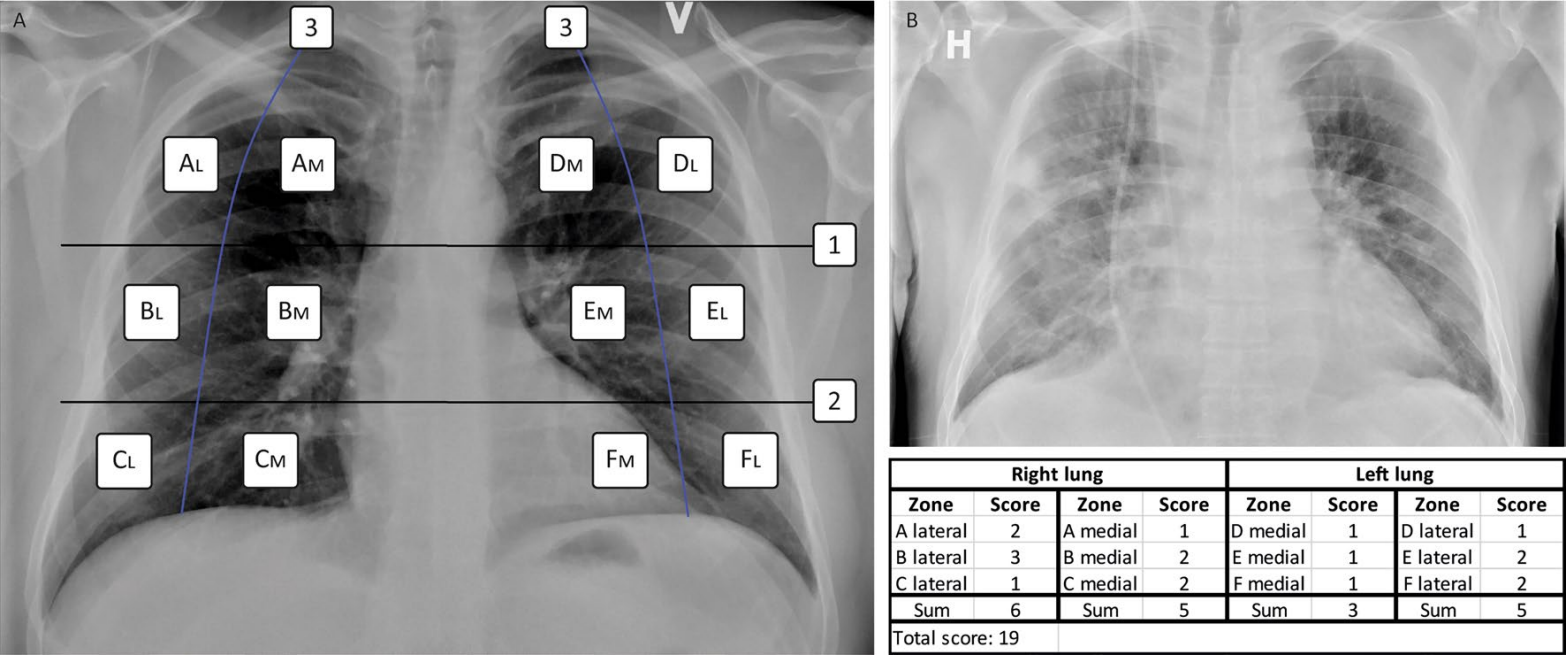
(A) The MBrixia scoring system. Box 1 and 2 mark the horizontal division corresponding to the inferior wall of the aortic wall, and the inferior wall of the right pulmonary vein, respectively, creating three zones in each lung: A, B, C, D, E and F. Box 3 marks the vertical line drawn from the pulmonary apices to the diaphragm, creating further division into medial (M) and lateral (L) zones. (B) A clinical example of a chest x-ray image scored using the MBrixia score.
